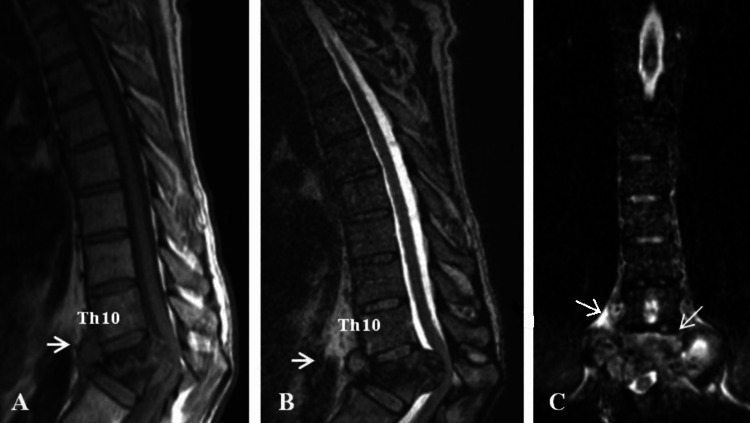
MRI of the tuberculous spondylodiscitis at the level of T11 – T12 (Case 2) (A) Sagittal projection T1 – heterogeneous hypointense signal with pronounced bone marrow edema, subligamentous spread of the infection, with pronounced compression of the spinal cord and paravertebral abscess (thin solid arrow); (B) Sagittal projection T2 shows heterogeneous hyperintense signal, compression of the spinal cord and paravertebral abscess (thin solid arrow); (C) Coronal projection T2 stir visualizes well the paravertebral abscess around the affected segments (thin solid arrows).
